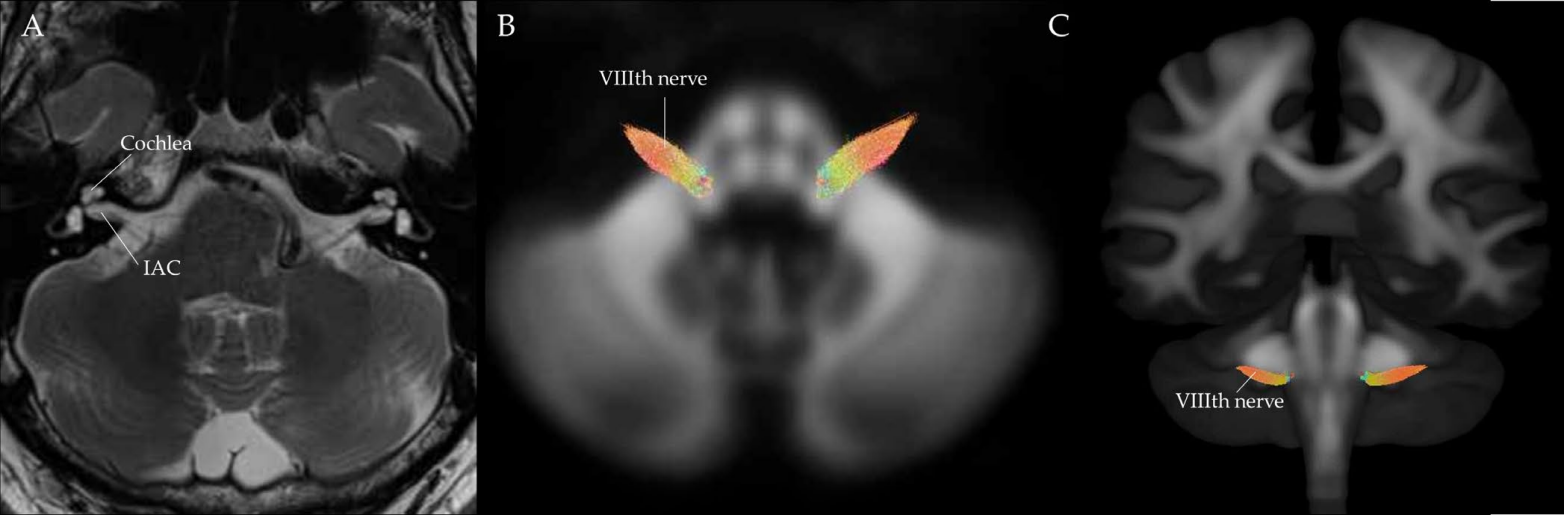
(A) T2‐weighted MR image showing an axial slice acquired from a participant. The cochlea and internal auditory canal (IAC) are clearly identifiable. (B) White matter fibre orientation distribution function population tempate, axial slice, with the VIIIth nerves shown. The VIIIth nerves were generated with probabilitic tractography using the dMRI data. (C) Coronal view of the same image shown in (B). The position of the VIIIth nerves are shown.
